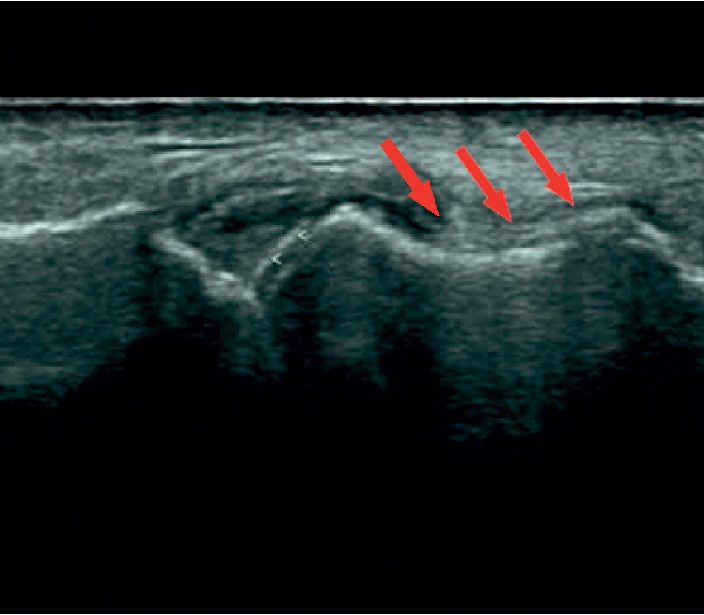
Functional ultrasound scan of the knee.
